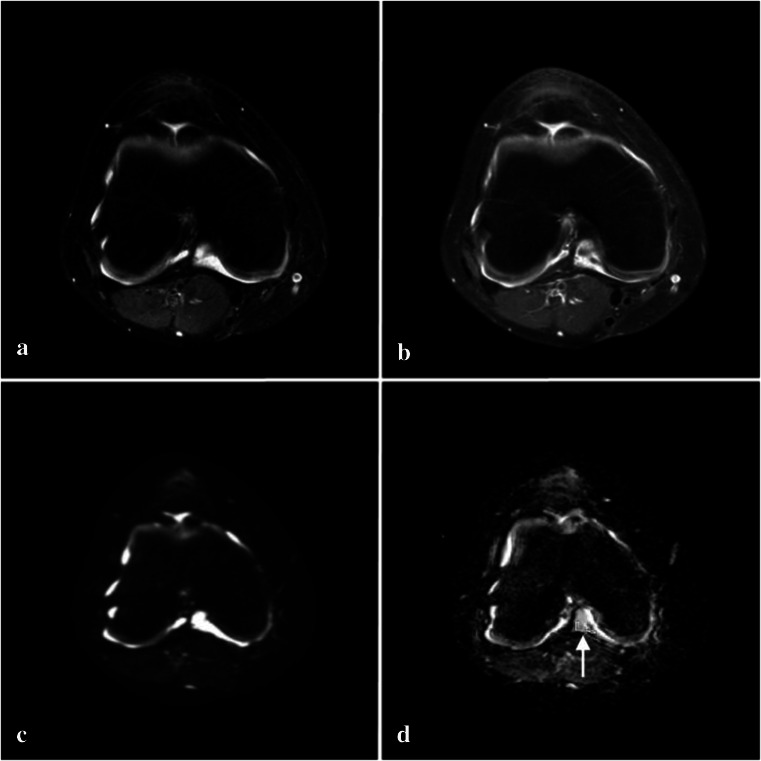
MRI of the knee of an 11-year-old female JIA patient. ROI selection of synovium on ADC_50–600_ map (*arrow points*
